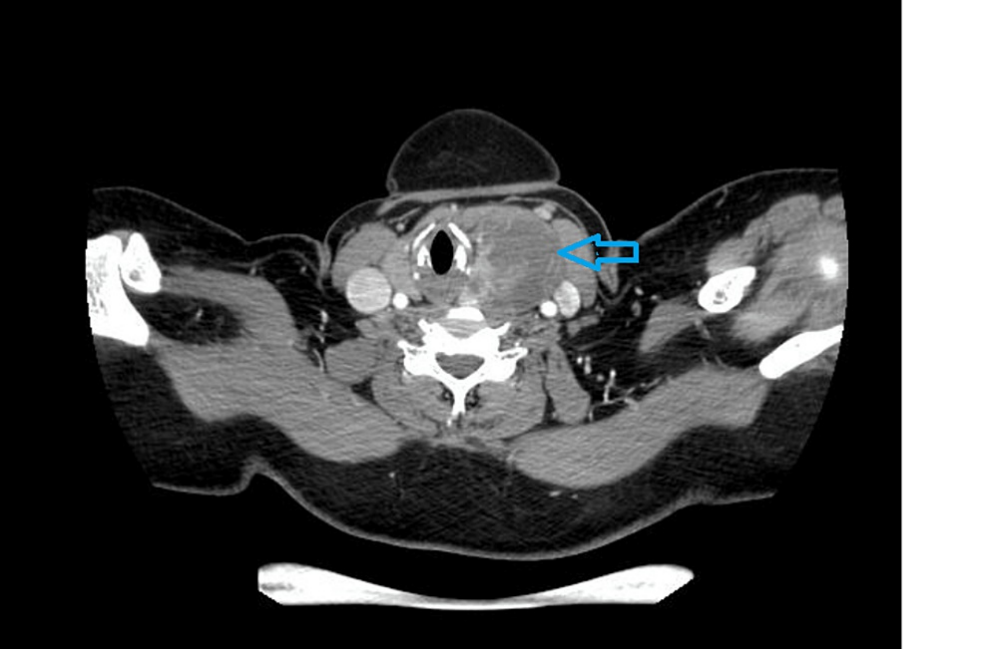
CT neck showing a cystic lesion of size measuring 5.2x4.5x3.4cm abutting the left lobe of thyroid with internal septations.
